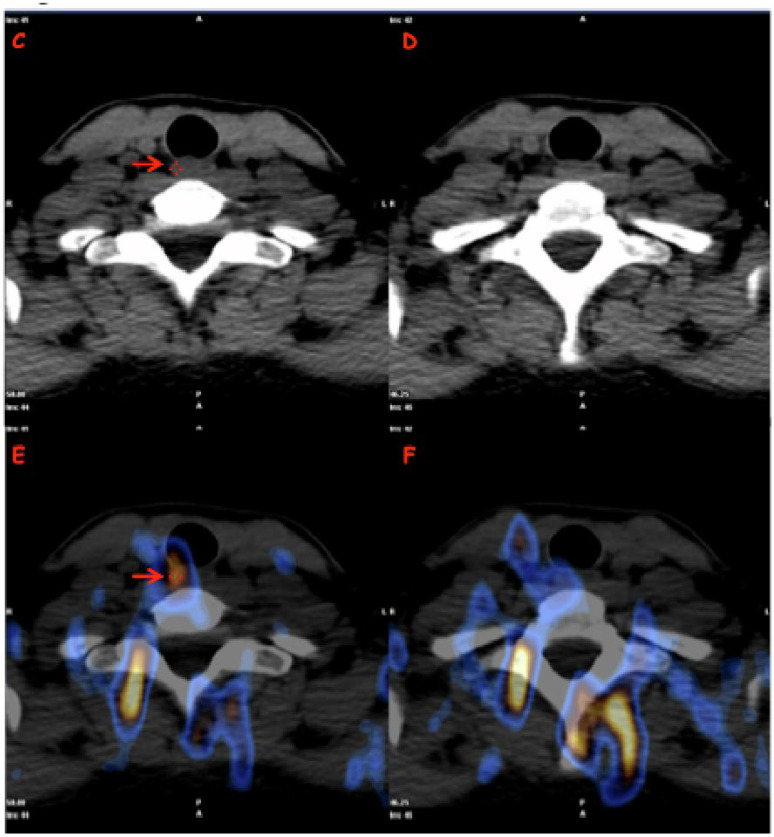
Forty year old woman, pT1b, Nx, Mx-PTC (classic variant), multifocal and bilateral. The largest malignant nodule (14 mm in size) was located in the lower part of the right lobe. (A) Dx-WBS (anterior and posterior views) and (B) static images (anterior and posterior views) of the neck-thoracic region were obtained 3 h after iodine-123 administration (259 MBq after rhTSH stim-ulation). No pathological radioiodine uptake was noted. On the contrary, axial SPECT-CT images (C–F) showed abnormal radioiodine uptake in a small para-tracheal lymph-node (red arrows) of the right central compartment (VI Robbins’ level). At the time of 123I-Dx-WBS-SPECT/CT study, nUs did not show any lymph-node with suspected/pathological features. Basal-Tg was 0.9 ng/mL while early and late-stimulated-Tg levels were <10 ng/mL (2.7 and 7.7 ng/mL, respectively). The patient under-went functional lymphadenectomy of the right central compartment and histophatological analysis confirmed the presence of micrometastasis in 4 out of 13 removed lymph-nodes.
